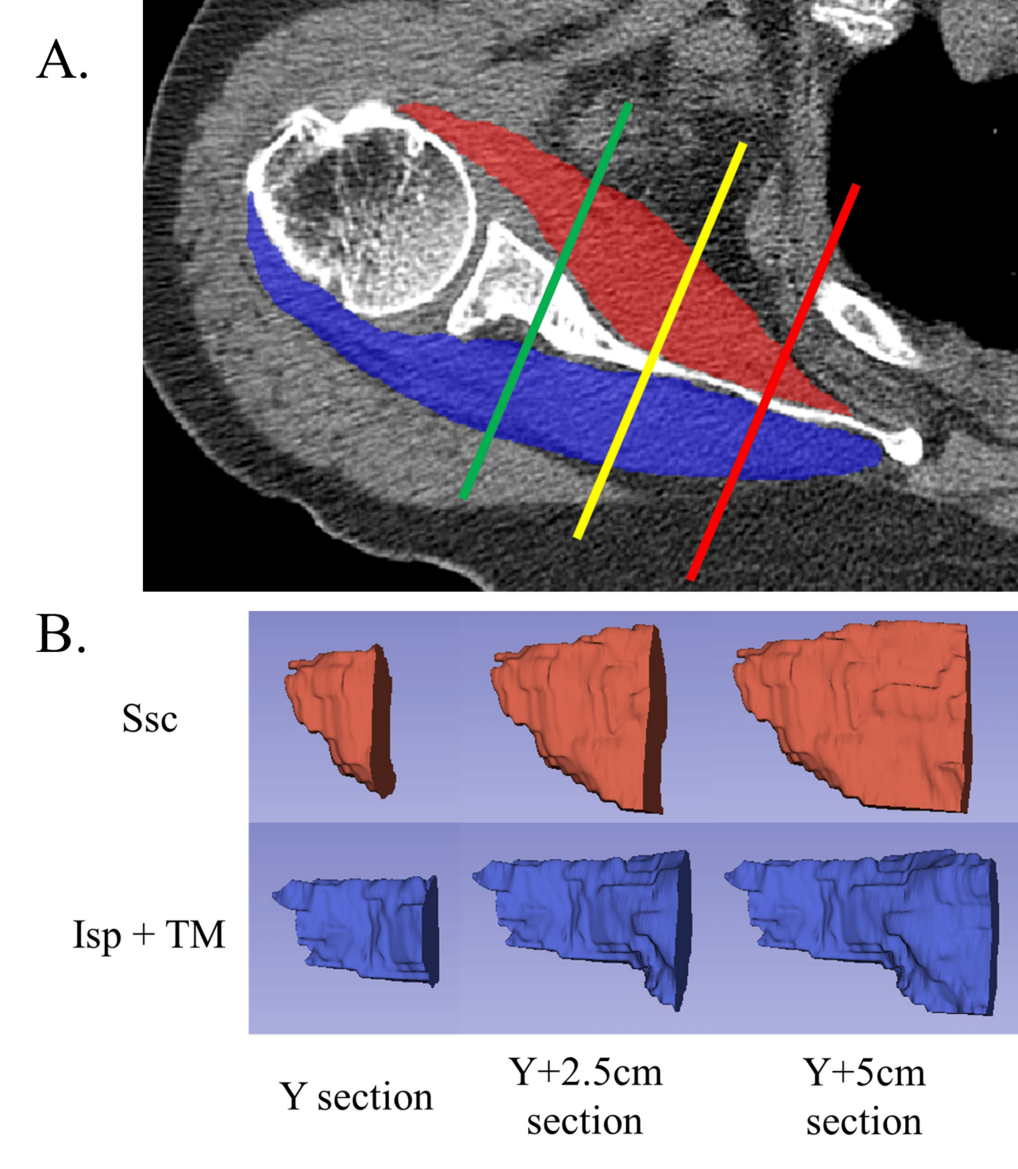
Measurement of the volume and CT density of the shoulder muscle. (A) The areas of the subscapularis muscle (Ssc, red area) and infraspinatus and teres minor muscles (Isp+TM, blue area) in every axial image are delineated using semi-automatic segmentation tools. The three-dimensional (3D) models of the Ssc and Isp+TM are separated by the Y-view (Y) plane (green line), the Y+2.5 cm plane (yellow line), and the Y+5 cm plane (red line). Additionally, a cut-off was implemented at the plane 3 cm inferior to the lower end of the glenoid labrum (not shown in the figure) to exclude the inferior margin of each muscle volume, as this area is usually out of range in our shoulder CT protocol. (B) For each 3D model of the Ssc and Isp+TM muscles, three sections of 3D models were newly created (resulting in a total of six sectional muscle models): from the most lateral end of the muscles to the Y-view plane (i.e., Y section), from the plane 2.5 cm medial to the Y-view (i.e., Y+2.5 cm section), and from the plane 5 cm medial to the Y-view (i.e., Y+5 cm section). These images were created by the authors for this manuscript. CT, computed tomography.
